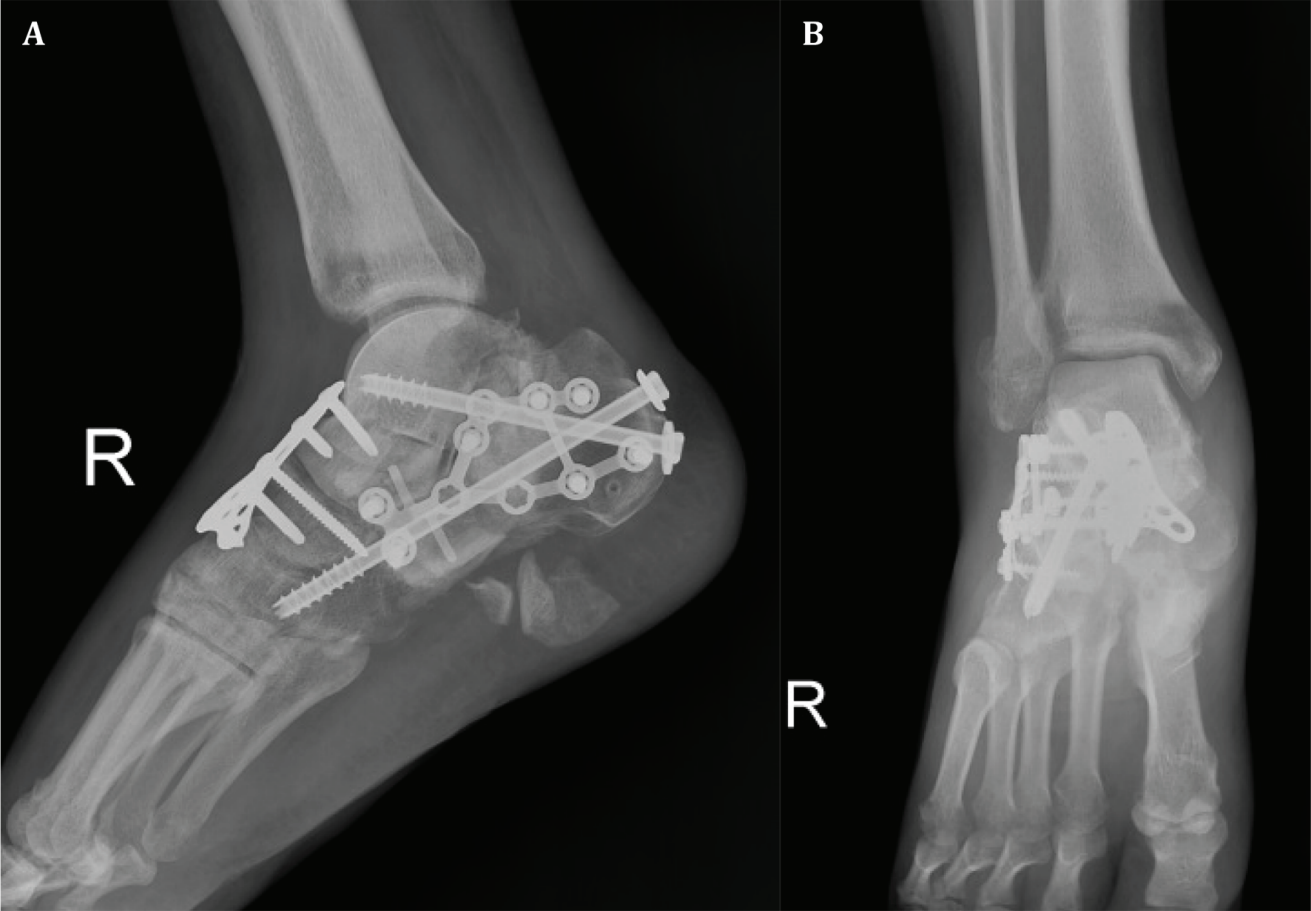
(A) Lateral and (B) anteroposterior radiographs of right ankle after the second surgery
